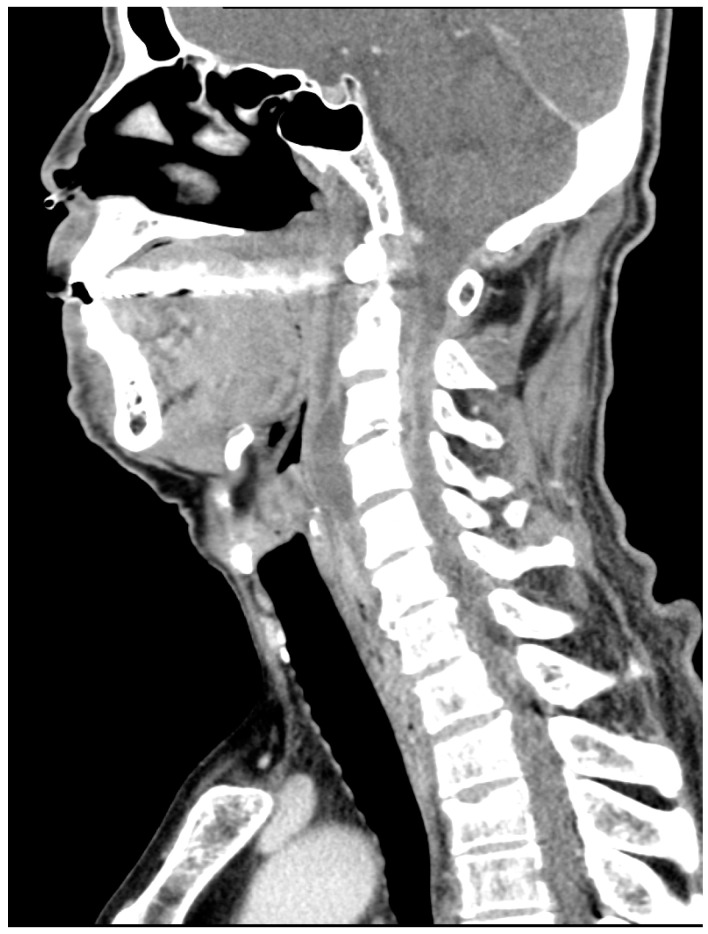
Enhanced cervical CT on admission (day 0) showing a low-density area with a peripheral enhancement effect. Vertebral body and adjacent soft tissue suggest C3/4 spondylosis and an abscess formation.
